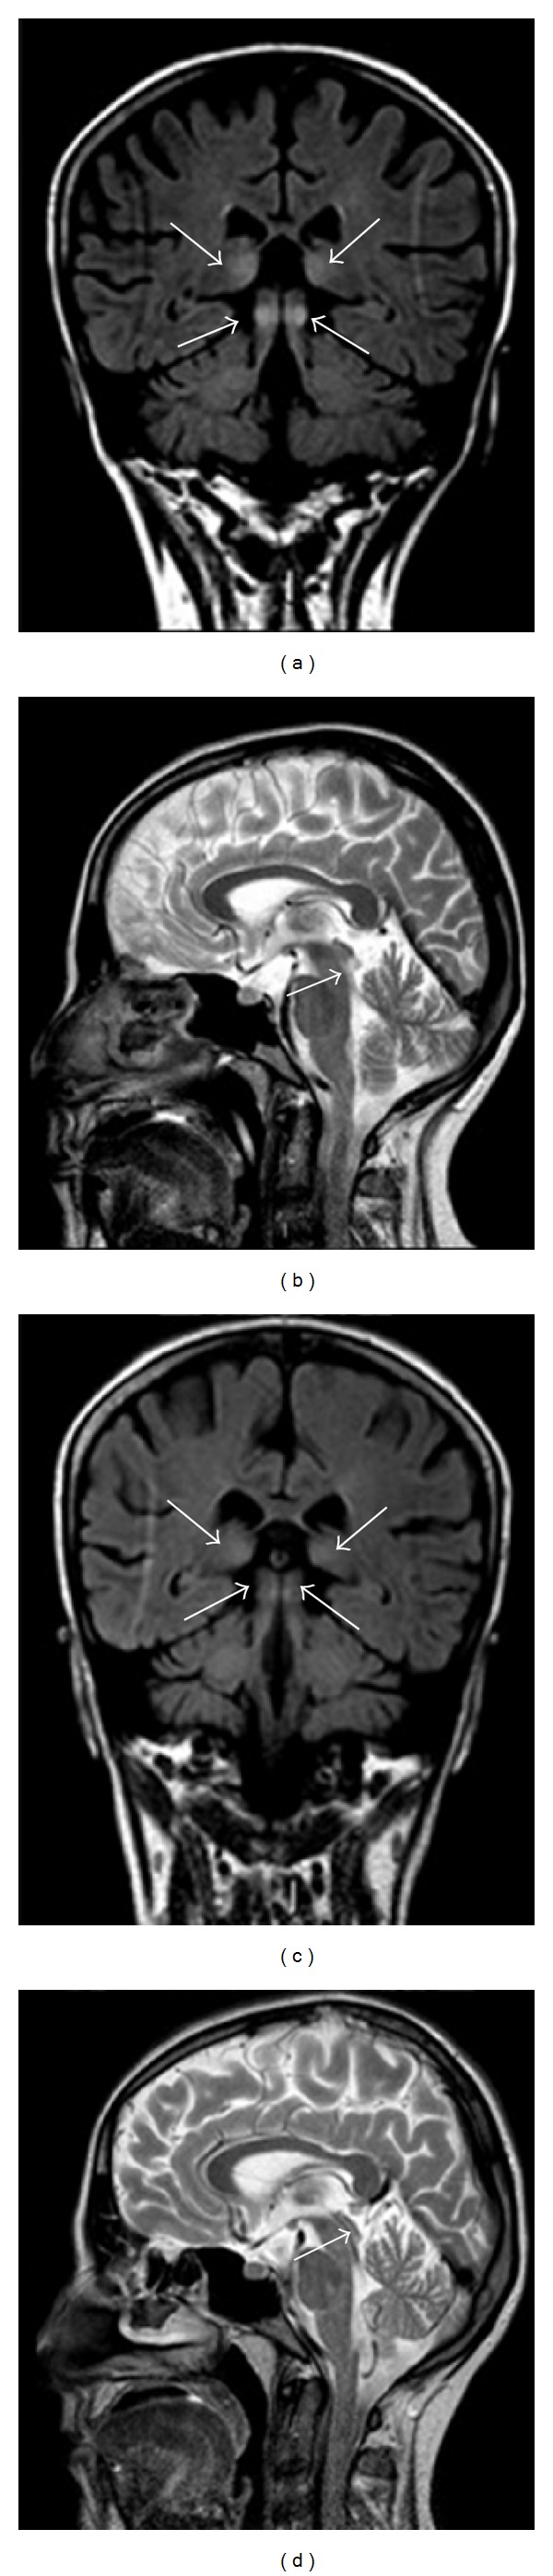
Thirty-three-year-old woman, primipara, in her 16th week of gestation, with a 3-week history of severe persistent nausea and vomiting accompanied by a 6 kg weight loss. FLAIR coronal (a) and T2-weighted sagittal (b) images showing bilateral and symmetric hyperintense signal alteration at the level of the medial portion of the thalami and of the lower portion of the tectal plate. A 3-week posttherapy (thiamine administered 100 mg/day intravenously for 10 days then 300 mg/day orally) brain MRI showed partial resolution of the signal abnormalities previously observed (c-d).
